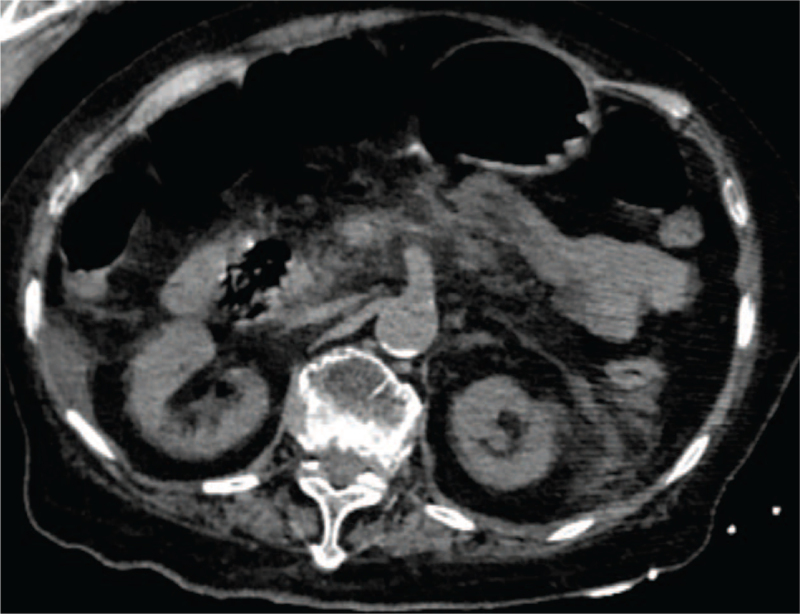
Abdominal and pelvic computed tomography (CT) revealed that an irregular pancreatic shape in the abdominal cavity, with the normal pancreatic duct. Blurred fat space around the pancreas, and there was little effusion in the abdominal and pelvic cavity.
